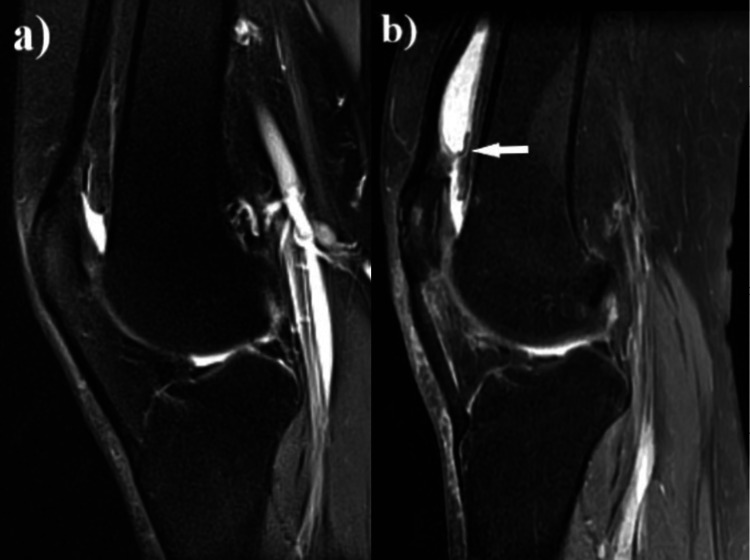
MRI images of the patient a) MRI performed for patellar tendonitis before the first operation. No pathology detected in the suprapatellar region, except no-passage of synovial fluid to the suprapatellar area; b) MRI image of the mass in the suprapatellar pouch before the re-arthroscopy. Liquid intensity mass isolated from the joint cavity by a band-like structure (arrow).
